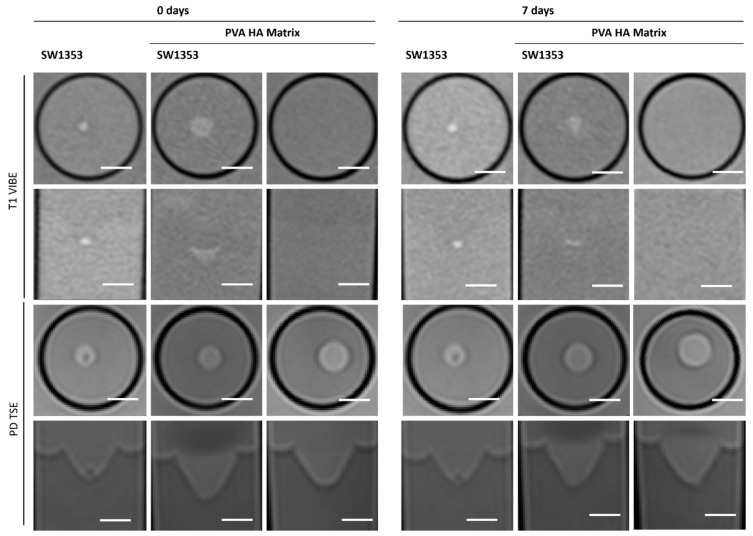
Cross-sectional (1st and 3rd row) and longitudinal axis (2nd and 4th row) images of tubes with SW1353 spheroids with and without PVA HA hydrogel and just PVA HA hydrogel. T1-weighted images (1st and 2nd row) were obtained using a spoiled gradient echo VIBE sequence. PD-weighted images were obtained using a TSE sequence. Scale bars represent 10 mm.
